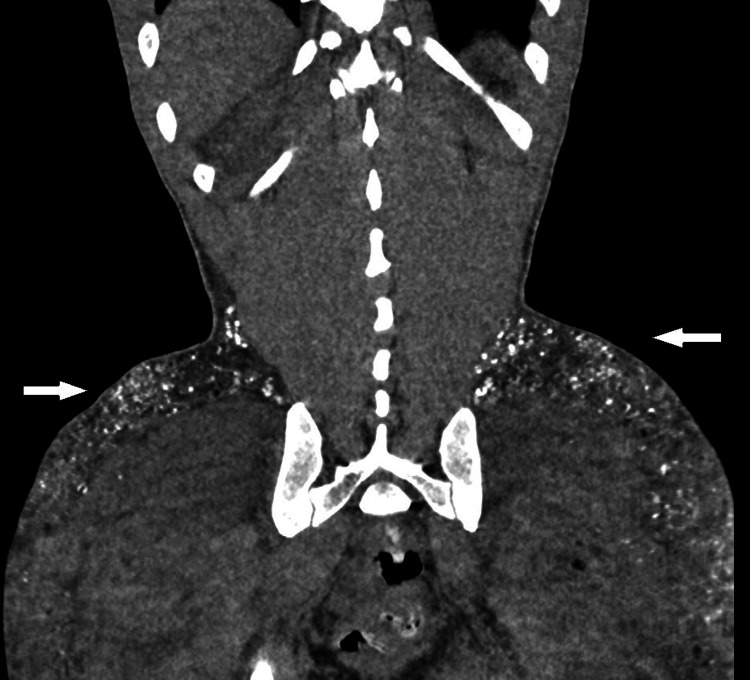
Coronal view of a computed tomography image from the chest to the proximal thighs, showing diffuse calcification around the gluteus and thighs bilaterally
